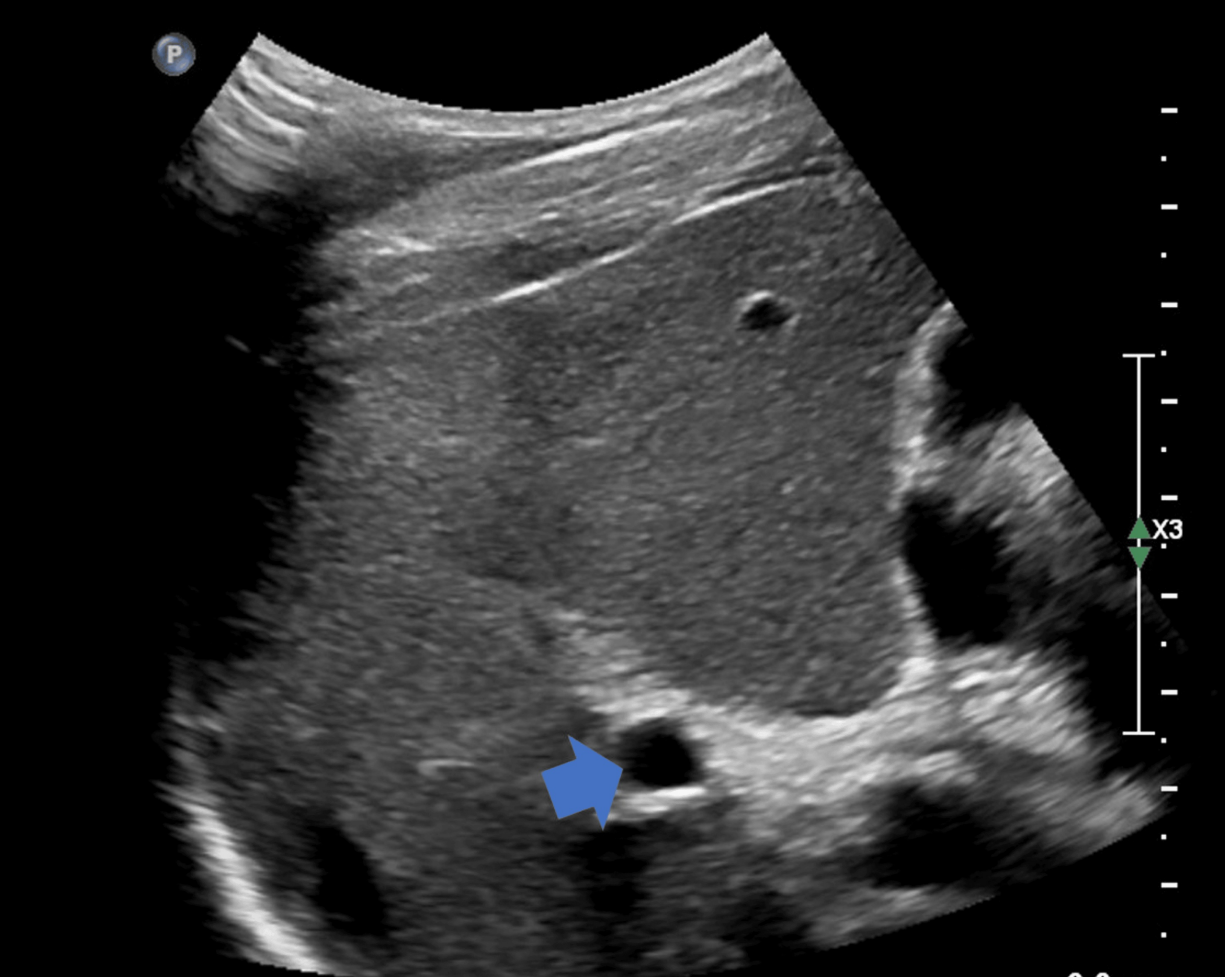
Ultrasound of the liver revealing a prominent portal vein (blue arrow) and heterogeneous liver echoes
